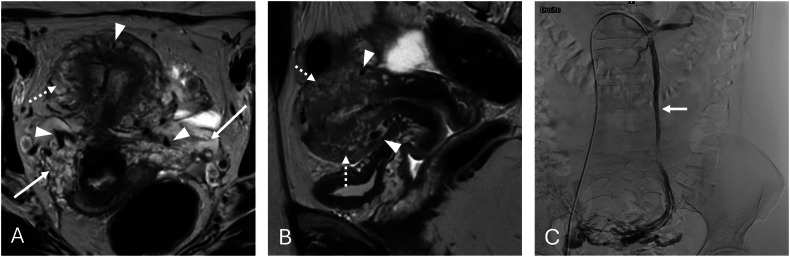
Pelvic congestion syndrome (PCS) in a 34-year-old patient presenting with dysmenorrhoea, dyspareunia, and pelvic pain, initially screened for endometriosis. A Axial and B sagittal T2-W MR images show diffuse T2-hyperintense venous varicosities of the outer myometrium (dotted arrows) with preservation of the zonal anatomy and normal appearance of the junctional zone, as well as predominantly left-sided venous varicosities in the parametria (arrows). The presence of some flow-voids (arrowheads) indicates faster venous flow. C Coronal image of venography with Valsalva manoeuvres demonstrates venous reflux into the left gonadal vein (arrow) associated with pelvic venous varicosities, confirming the clinical diagnosis of PCS
